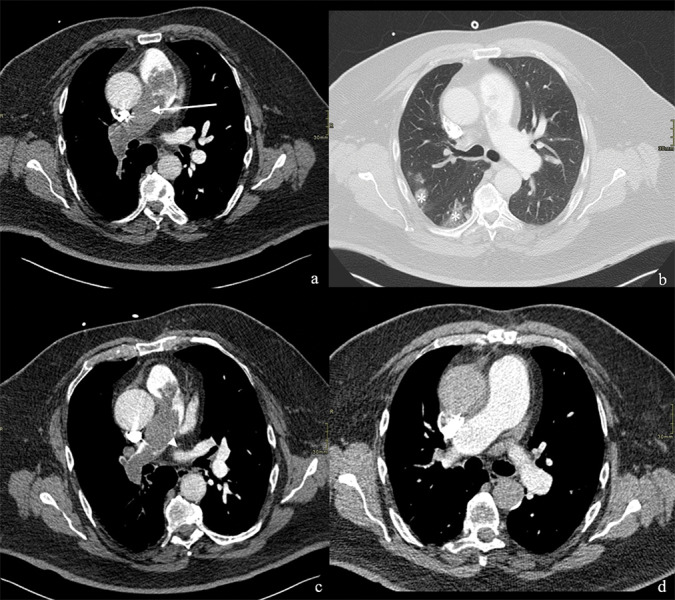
Axial CT pulmonary angiography. (a) Extensive intraluminal filling defects *(white arrow)* within the pulmonary trunk and right pulmonary artery. (b) Associated Hampton humps *(white asterisks)* in the right upper and lower pulmonary lobes, indicating pulmonary infarction. (c) One-week post-thrombolysis imaging demonstrating additional left pulmonary artery involvement *(white arrowhead)*. (d) Three months post-endarterectomy imaging showing no evidence of residual tumour.
